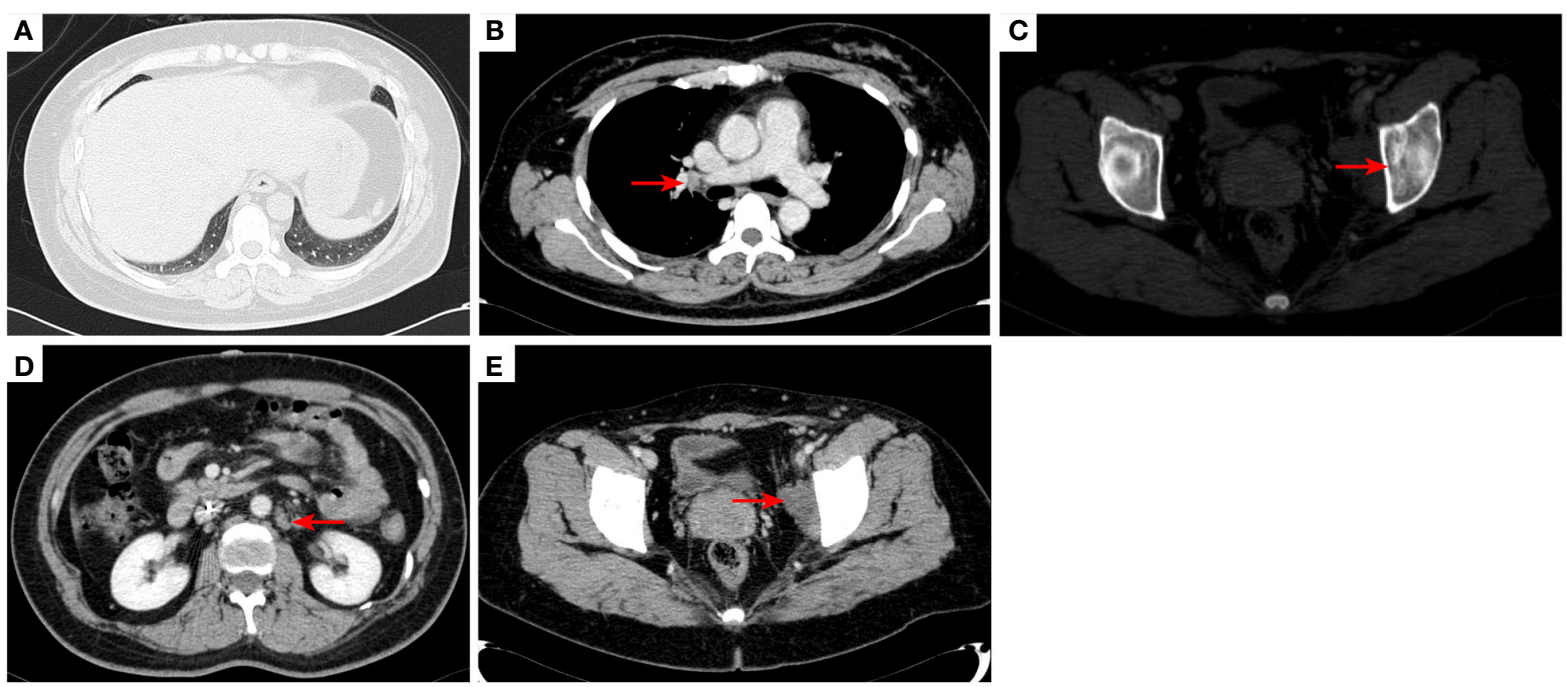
2020–11-3 CT scan: The original right lower lobe of the lung metastatic tumor disappeared (A); short diameter of new right hilar lymph node was 0.83 cm [red arrow, (B)]; Left acetabular metastases (red arrow, (C)). The short diameter of retroperitoneal lymph nodes was 1.01 cm which was bigger than before [red arrow, (D)], and the short diameter of left pelvic lymph nodes was 2.76 cm [red arrow, (E)]. The efficacy was PD. PD, progressive disease.
